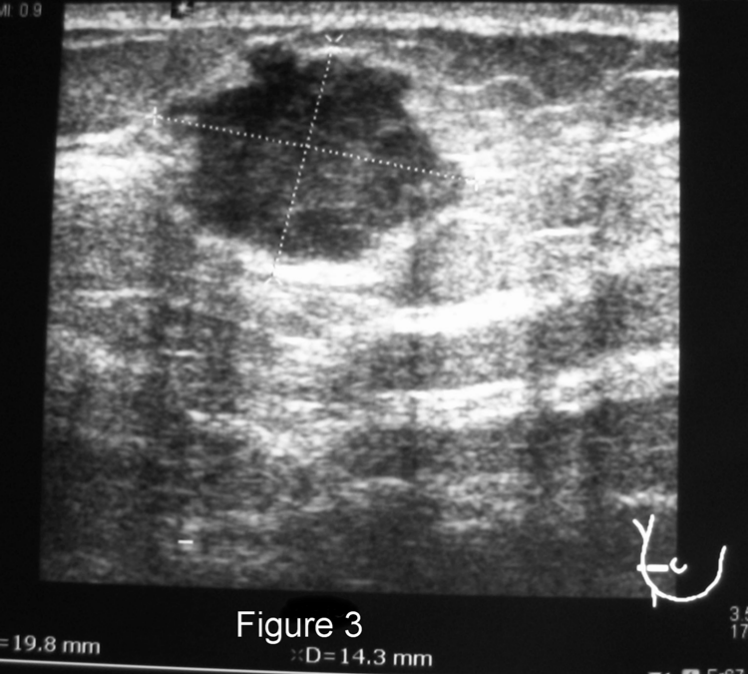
Right breast ultrasound from a 35 year-old patient with bilateral palpable breast masses. The ultrasound documented a 19 × 14 mm hypoechoic mass. She was found to have metastatic disease to her breast from a lung neuroendocrine carcinoma.
